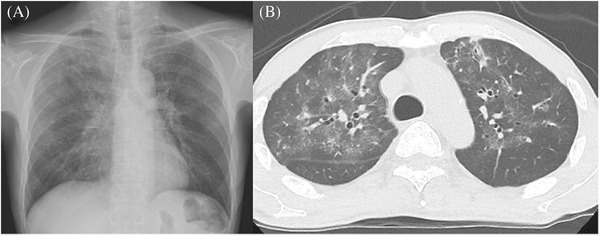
(A) Chest radiography and (B) computed tomography revealed the presence of bilateral ground glass opacities affecting mainly the upper lobes
